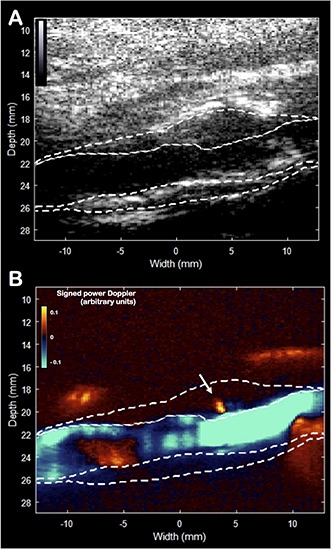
Ultra-sensitive Doppler imaging. B-mode picture (A) and the signed power Doppler picture (B). The plaque was manually delineated. The white arrow indicates the presence of an isolated centrifugal micro-flux originating from the lumen inside the plaque’s wall, which may correspond to a rupture zone of the fibrous cap.
